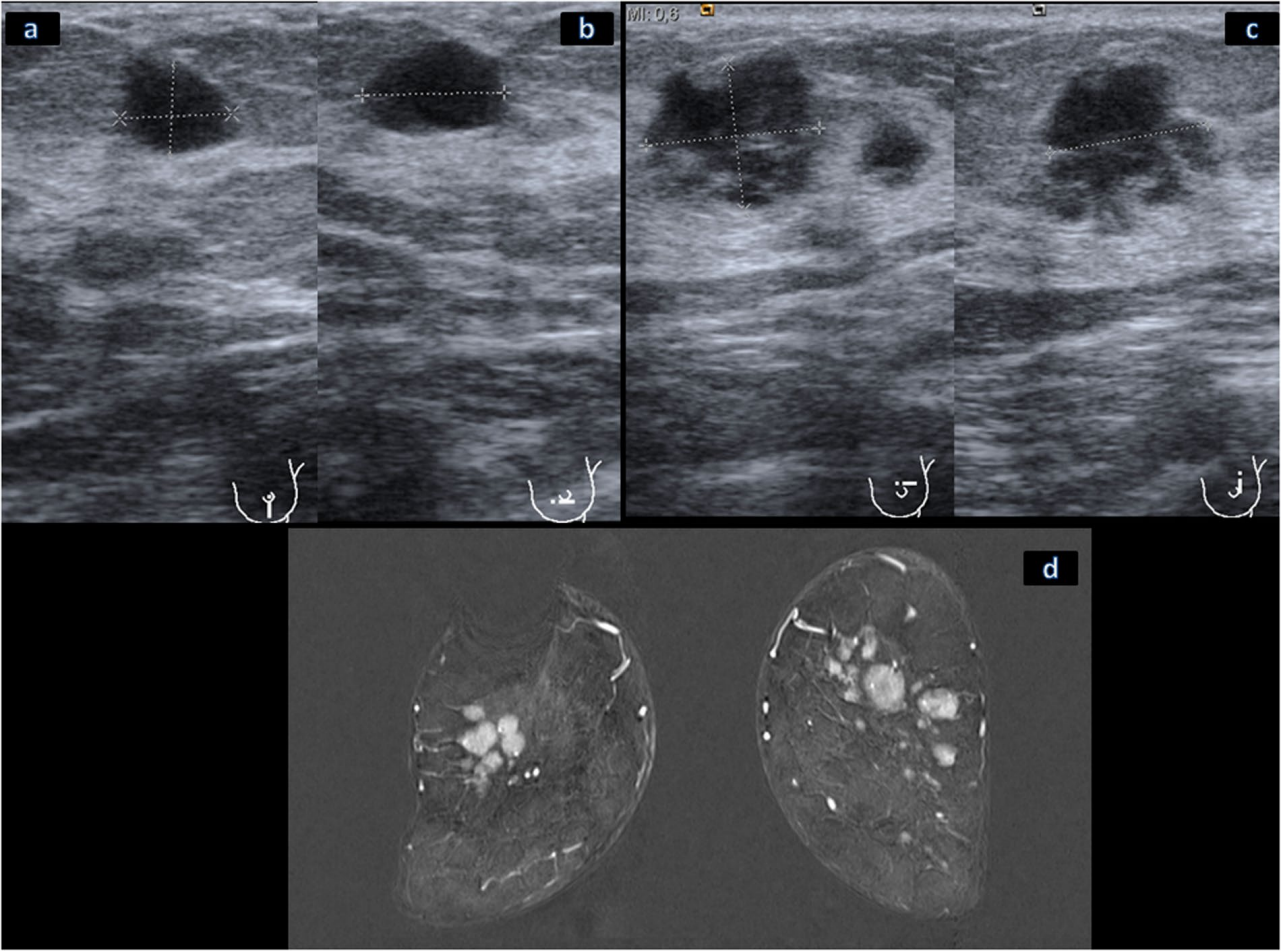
Ultrasound imaging findings in a 64-year old woman with breast T-cell lymphoma. The horizontal (a) and vertical (b) imaging planes demonstrated an oval, hypoechogenic mass with indistinct margins and without posterior acoustic features, located in the 6 o’clock position of the left breast. Another lesion in the upper outer quadrant of the left breast demonstrated in the vertical and horizontal imaging planes an irregular, complex cystic and solid mass with indistinct margins and with combined posterior acoustic pattern (c). The MRI imaging in coronal view (d) documented in the subtraction images multiple round and oval intramammary masses of both breasts with irregular margins and a homogeneous intensive enhancement.
